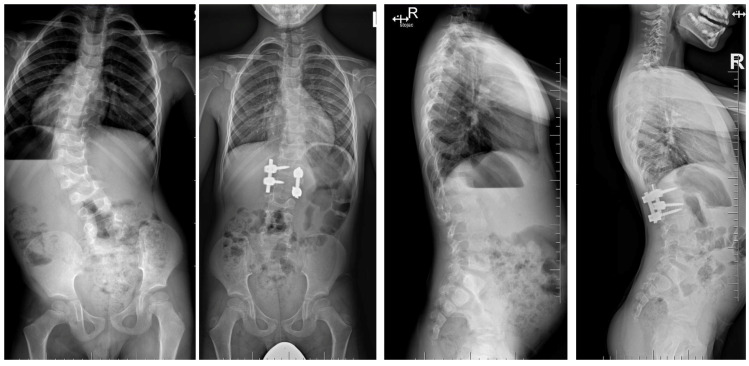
X-rays of 3-year-old boy with congenital scoliosis caused by additional hemivertebrae in thoracic and lumbar spine. Hemivertebrectomy was performed in lumbar spine from posteriori approach only with short posteriori spinal fusion. X-rays show spine before surgery and at 4-year follow-up.
